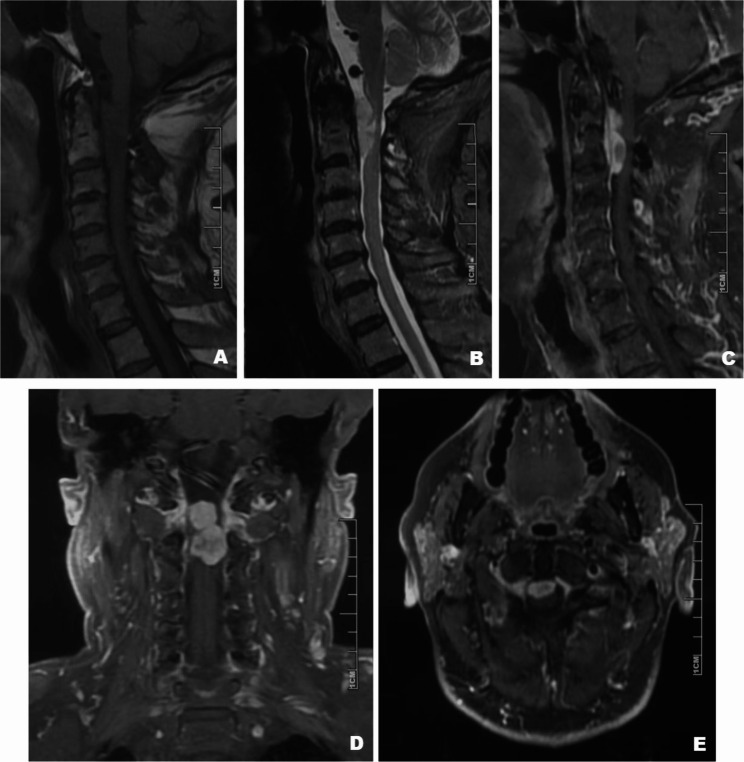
Preoperative MRI of the cervical spine demonstrating an extramedullary lesion at the C1–2 level. A Sagittal T1-weighted image shows isointensity; B Sagittal T2-weighted image reveals mixed hyperintensity; C–E Gadolinium-enhanced sagittal (C), coronal (D), and axial (E) T1-weighted images show heterogeneous contrast enhancement of the tumor
